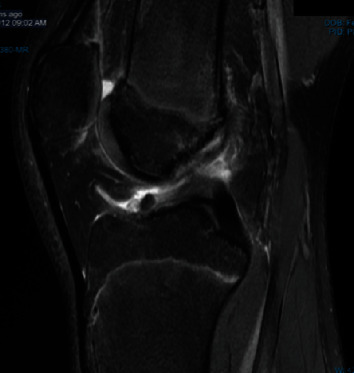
MRI demonstrating initial ACL rupture in 2012.
